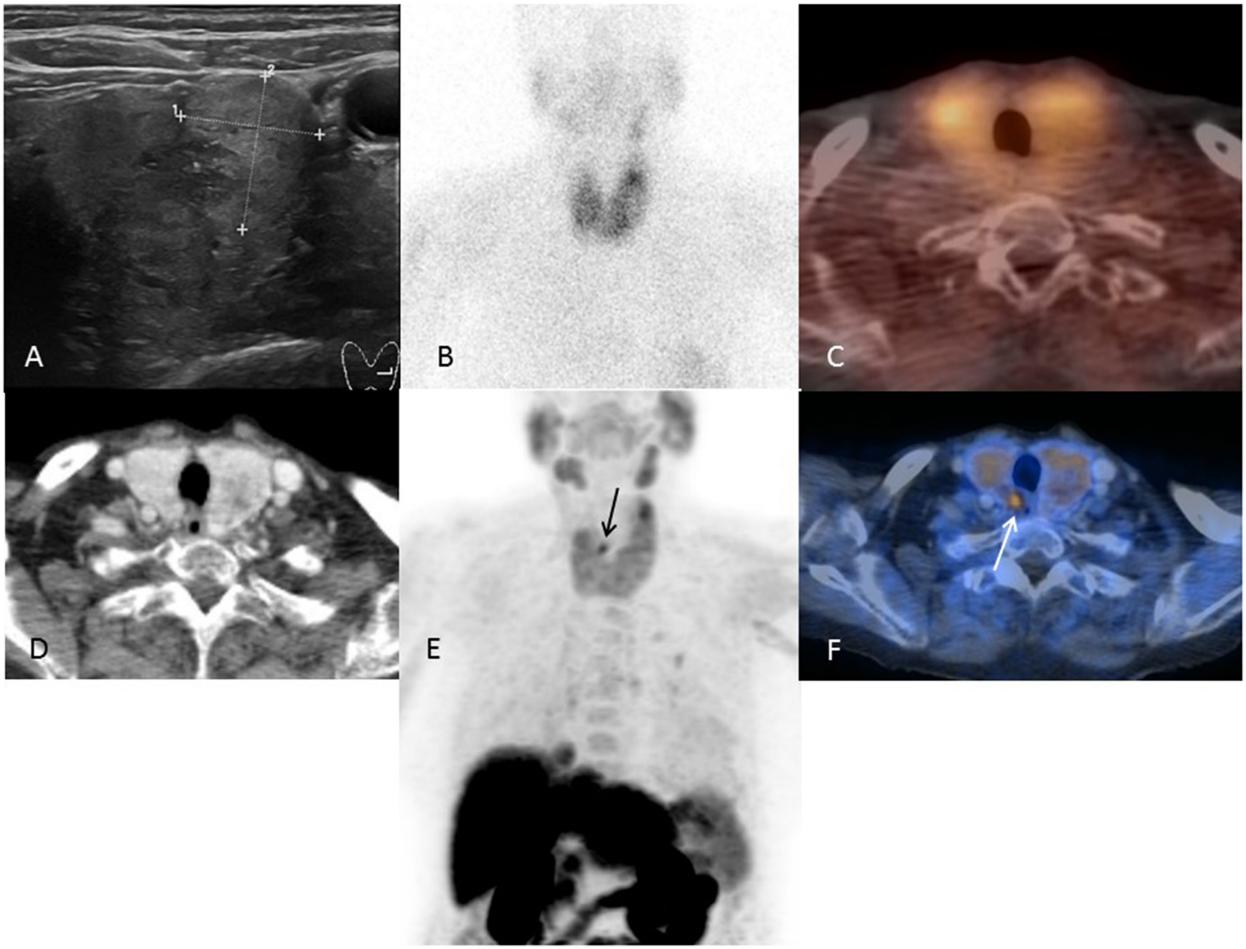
A representative case of a patient with multinodular goiter and negative conventional imaging. Images of an 82-year-old patient (patient number 9). Ultrasound (A) showing a bilateral multinodular goiter and no visible parathyroid adenoma. ^99m^Tc-sestamibi SPECT/CT (B,C) without detection of a parathyroid adenoma. FCH-PET/CT (D–F) with clear visualization of a small parathyroid retrotracheal adenoma at the upper right pole.
